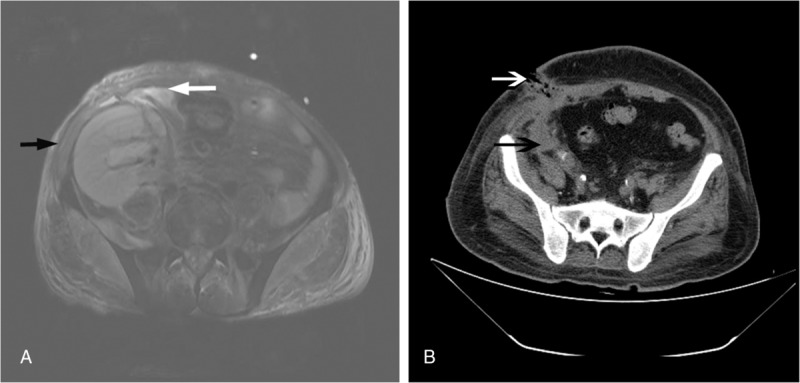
(A) Magnetic resonance imaging from patient 1 showed an enlarged renal graft, abdominal and pelvic soft tissues with stripe and grid high signal (black arrow), and pelvic peritoneal fluid signal (white arrow); which was suggestive of the transplanted kidney and perirenal tissue infection. (B) Computerized tomographic scanning of case 5 showed big low density shape shadow in the iliac fossa (black arrow) and soft tissue defects at the incision (white arrow), indicating massive fluid and incision infection.
